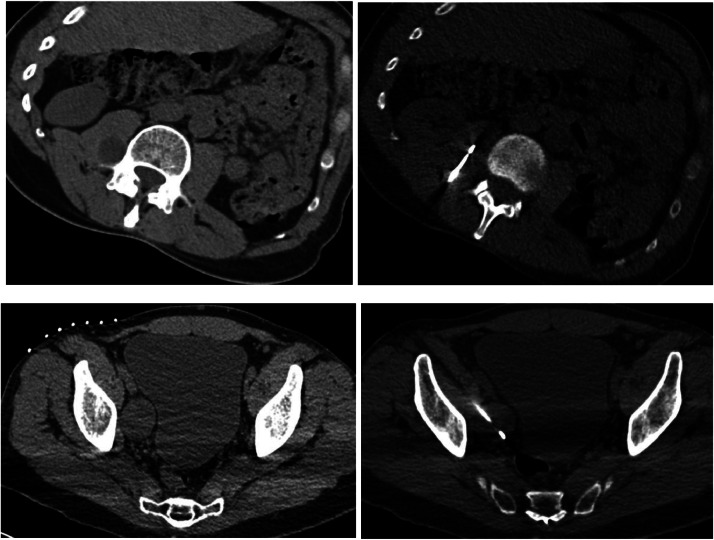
Axial CT images demonstrate the right psoas (A) and pelvic masses (C) and subsequent CT guided needle placement for biopsies of these lesions; right psoas mass (B) and pelvic mass (D).
